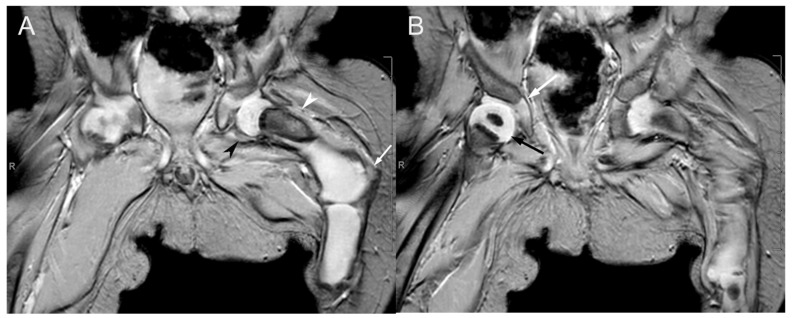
Coronal T2*-weighted MRI sequences of the proximal femora at one year of age. (A) The cartilaginous proximal left femoral epiphysis articulates with the left acetabulum. Only an oval-shaped ossified portion of the left femur is visible at the proximal femoral neck (hypointense region indicated by the white arrowhead). The remaining portions of the left femur are cartilaginous (hyperintense region indicated by the black arrowhead), with lateral bowing at the transition between the left femoral neck and the proximal diaphysis (white arrow). (B) The partially visualized right femur demonstrates normal articulation of the proximal femoral epiphysis (black arrow) with the right acetabulum (white arrow) and normal ossification of the proximal right femoral diaphysis.
